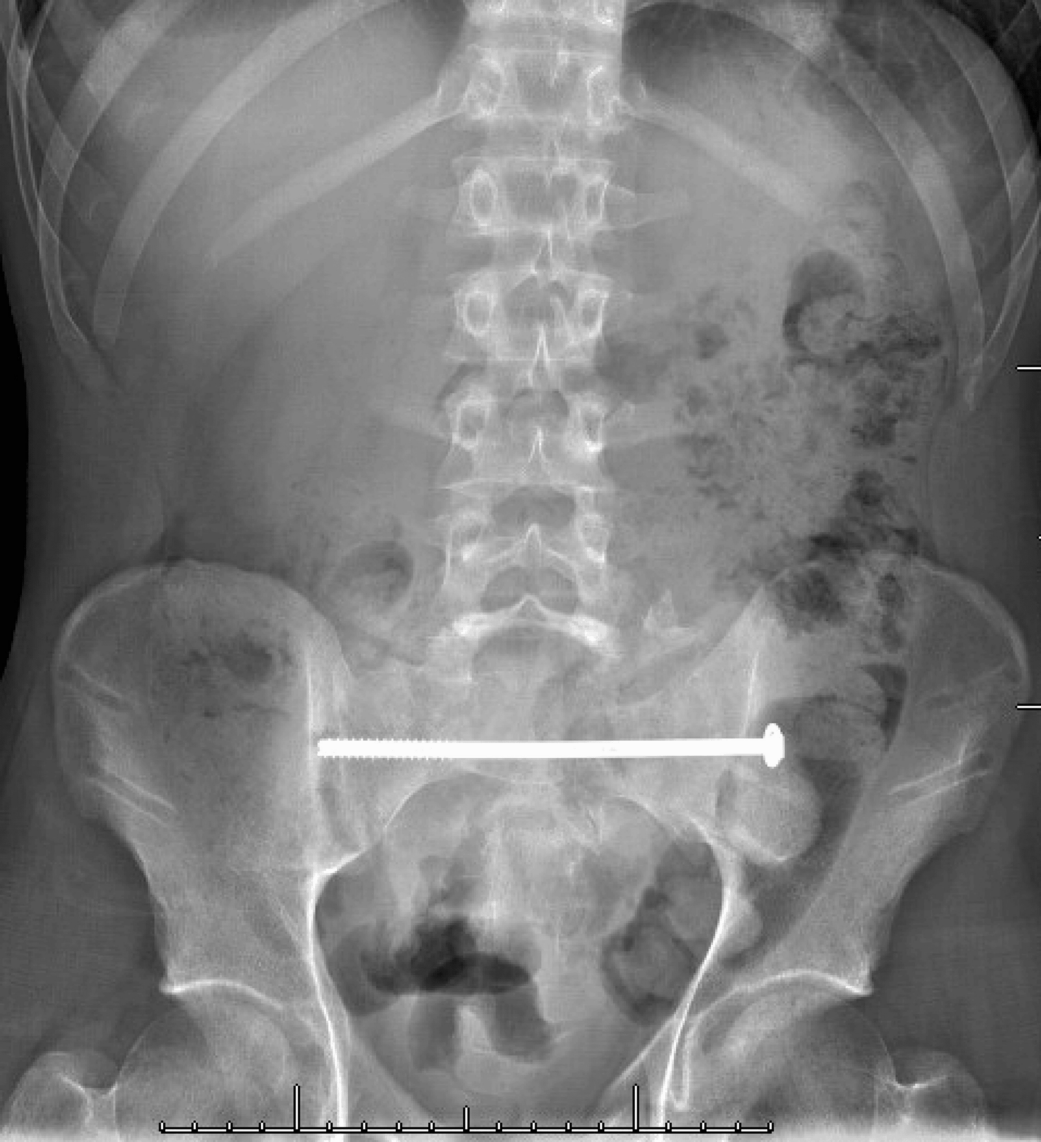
Initial Post-operative Sacral Screw Placement Anteroposterior X-ray from outside hospital demonstrating initial post-operative sacral screw placement
